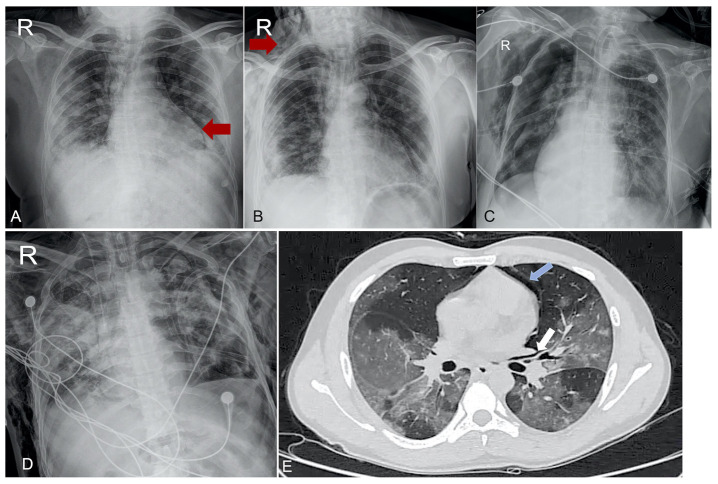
Radiographic characteristics of patients with COVID-19 ARDS. (A & B) A patient with COVID-19 ARDS developing spontaneous barotrauma beginning with pneumopericardium (A) and later progressing to subcutaneous emphysema (B). (C) A tracheostomized patient in the weaning mode developed bilateral pneumothorax requiring bilateral intercostal drain insertion. (D) A patient developed left-sided pneumothorax associated with bronchopleural fistula, for which two intercostal drains were inserted. The tracheal aspirate showed growth of Klebsiella pneumoniae. (E) High-resolution computed tomography of the chest showing peri-bronchial air (white solid arrow), indicative of positive Mackle’s sign and pneumopericardium (blue solid arrow).
